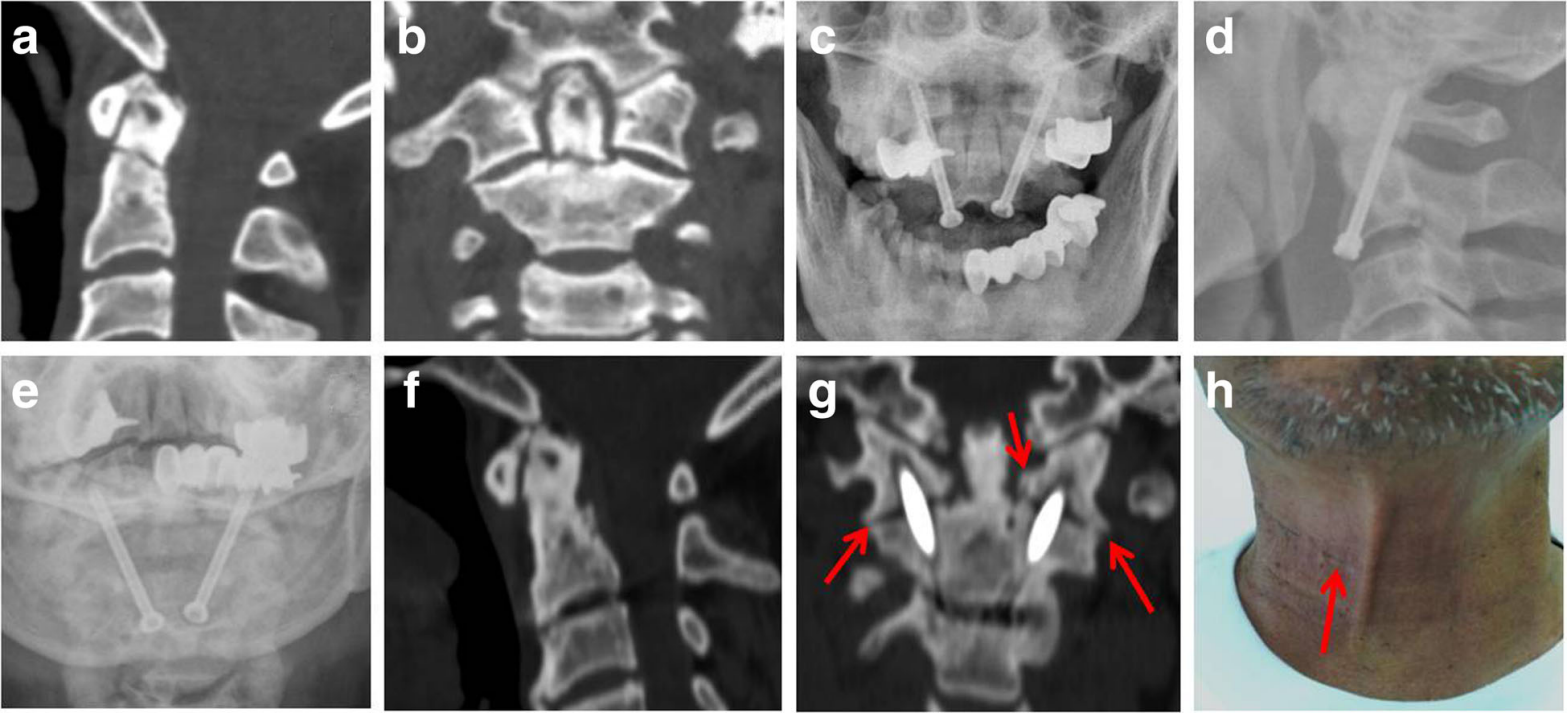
a, b The pre-operative 2D CT images of a 57-year-old man showed type II odontoid fracture. c, d Percutaneous anterior odontoid screw fixation failed, and percutaneous C1/2 transarticular screw fixation was performed on him as salvage technique. e Thirty-one months after operation, no screw loose or breakage was observed. f, g Bony fusion was observed at the odontoid fracture site, and bony fusion bridge was also observed at the C1/2 lateral articular facet on 2D CT images (g, Arrow); h only about 10 mm skin scar at his neck (arrow)
